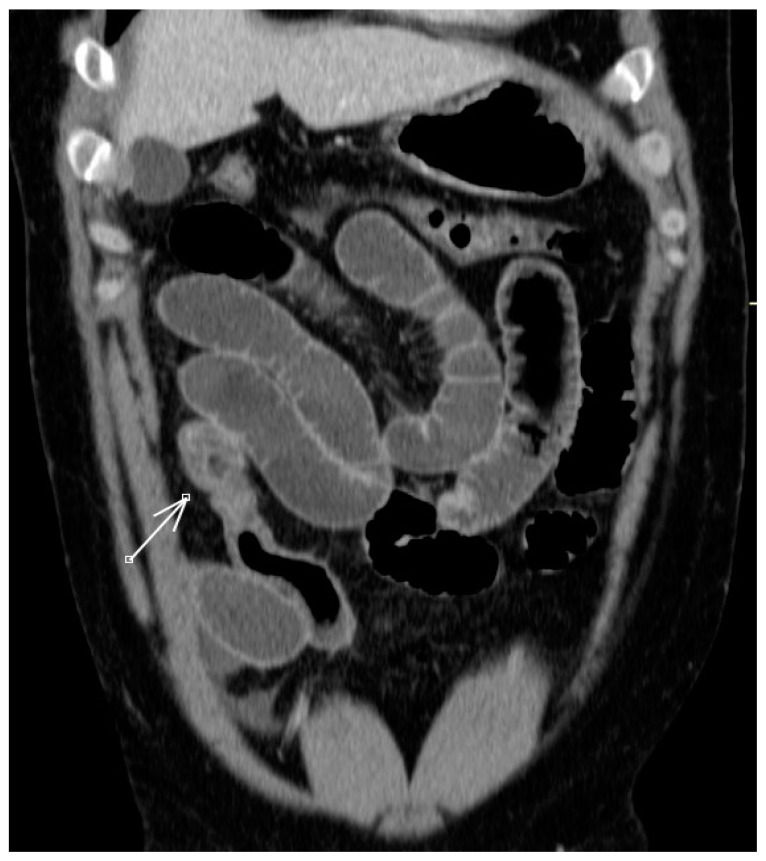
CT of abdomen coronal reconstruction: thickening of the wall of the loop of ileum, irregular morphology. The arrows represent asymmetric mural thickening of the intestinal wall with submucosal edema.
